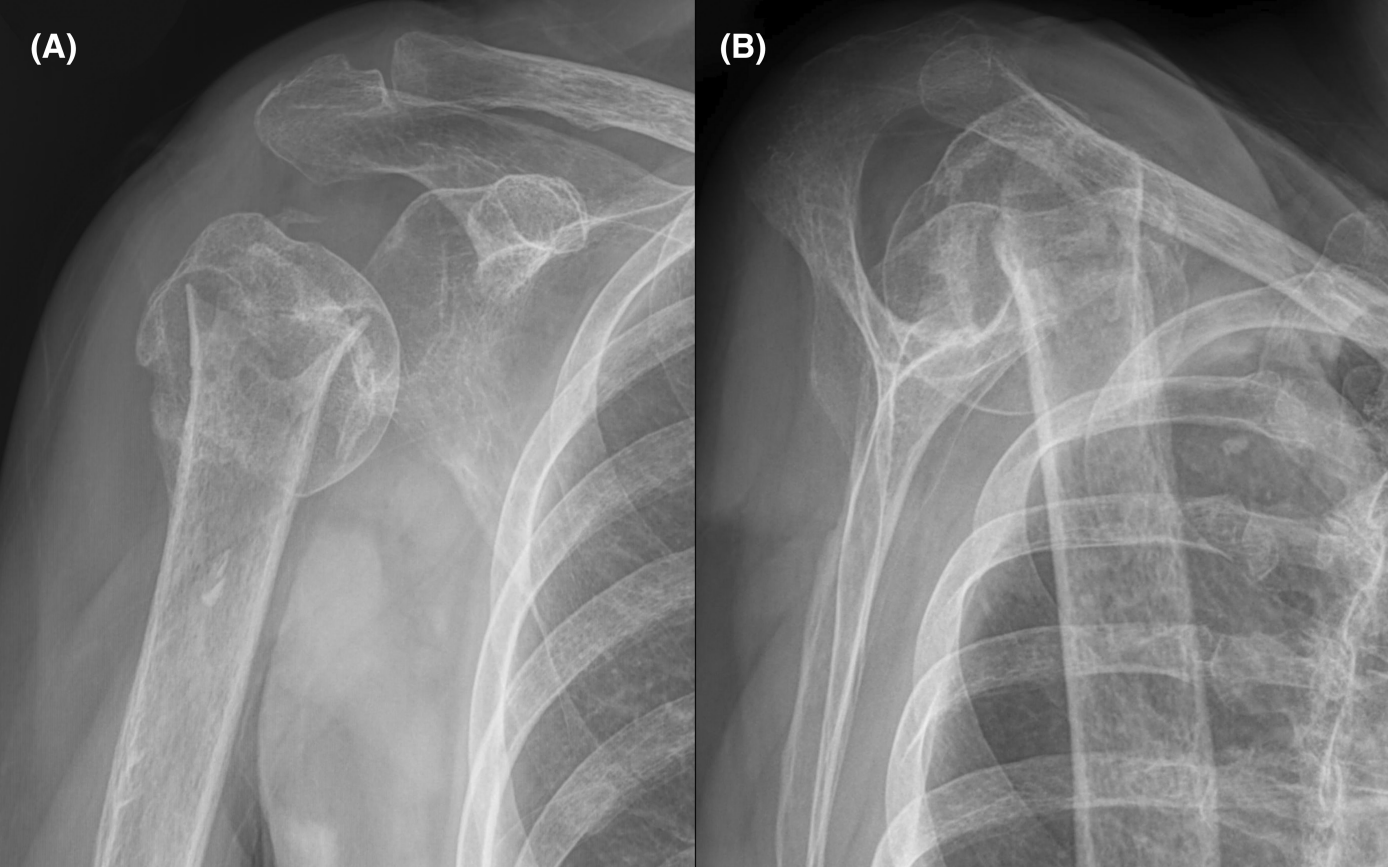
Anterior–posterior (A) and lateral (B) radiographs obtained at 4 months after the final debridement surgery. The fracture is clearly a displaced atrophic nonunion and a pseudarthrosis has formed.
